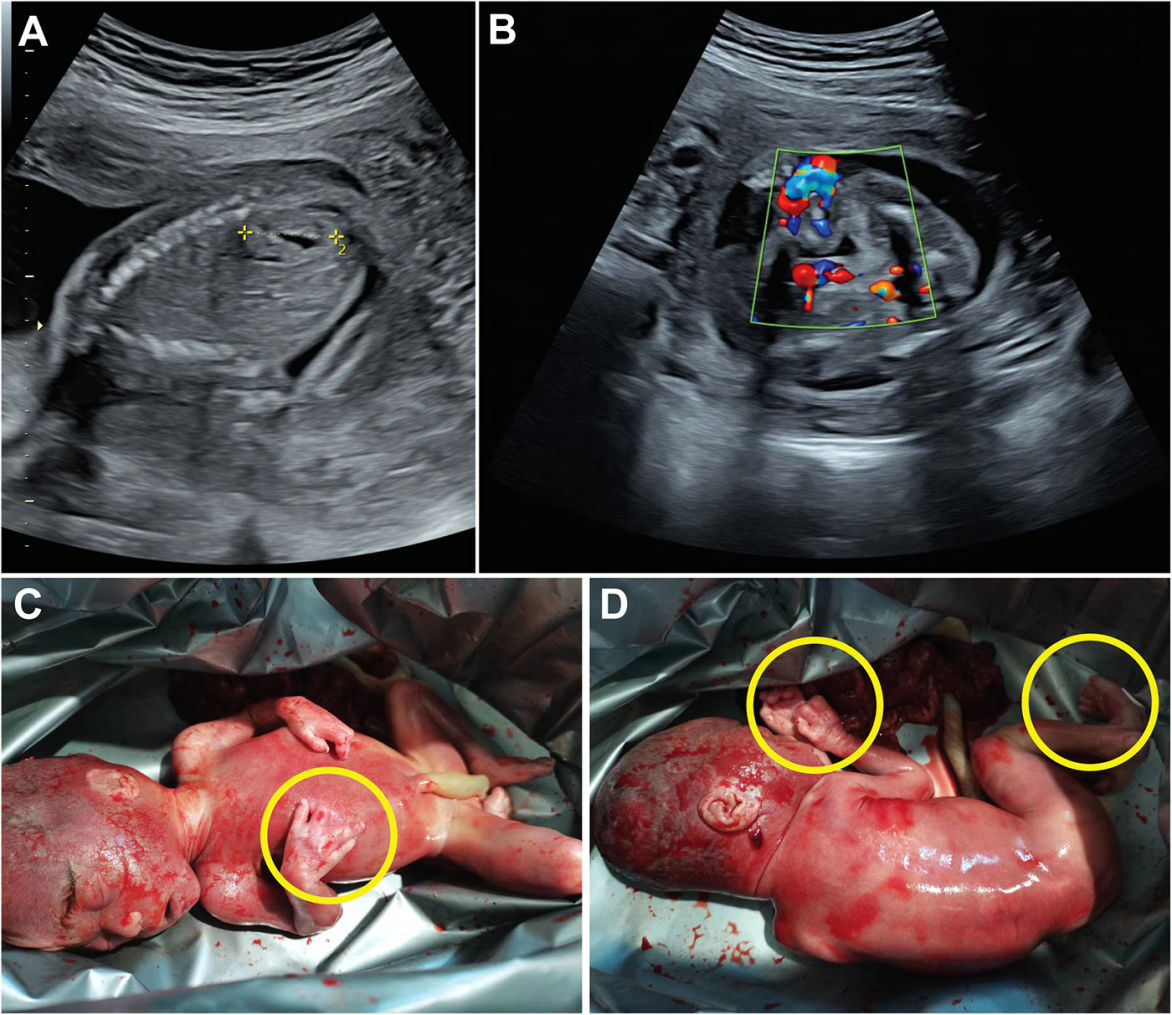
Phenotypic features of the fetus following termination of pregnancy. Ultrasound images labeled A and B show prenatal scans. Images C and D depict a newborn with yellow circles marking limb abnormalities.
